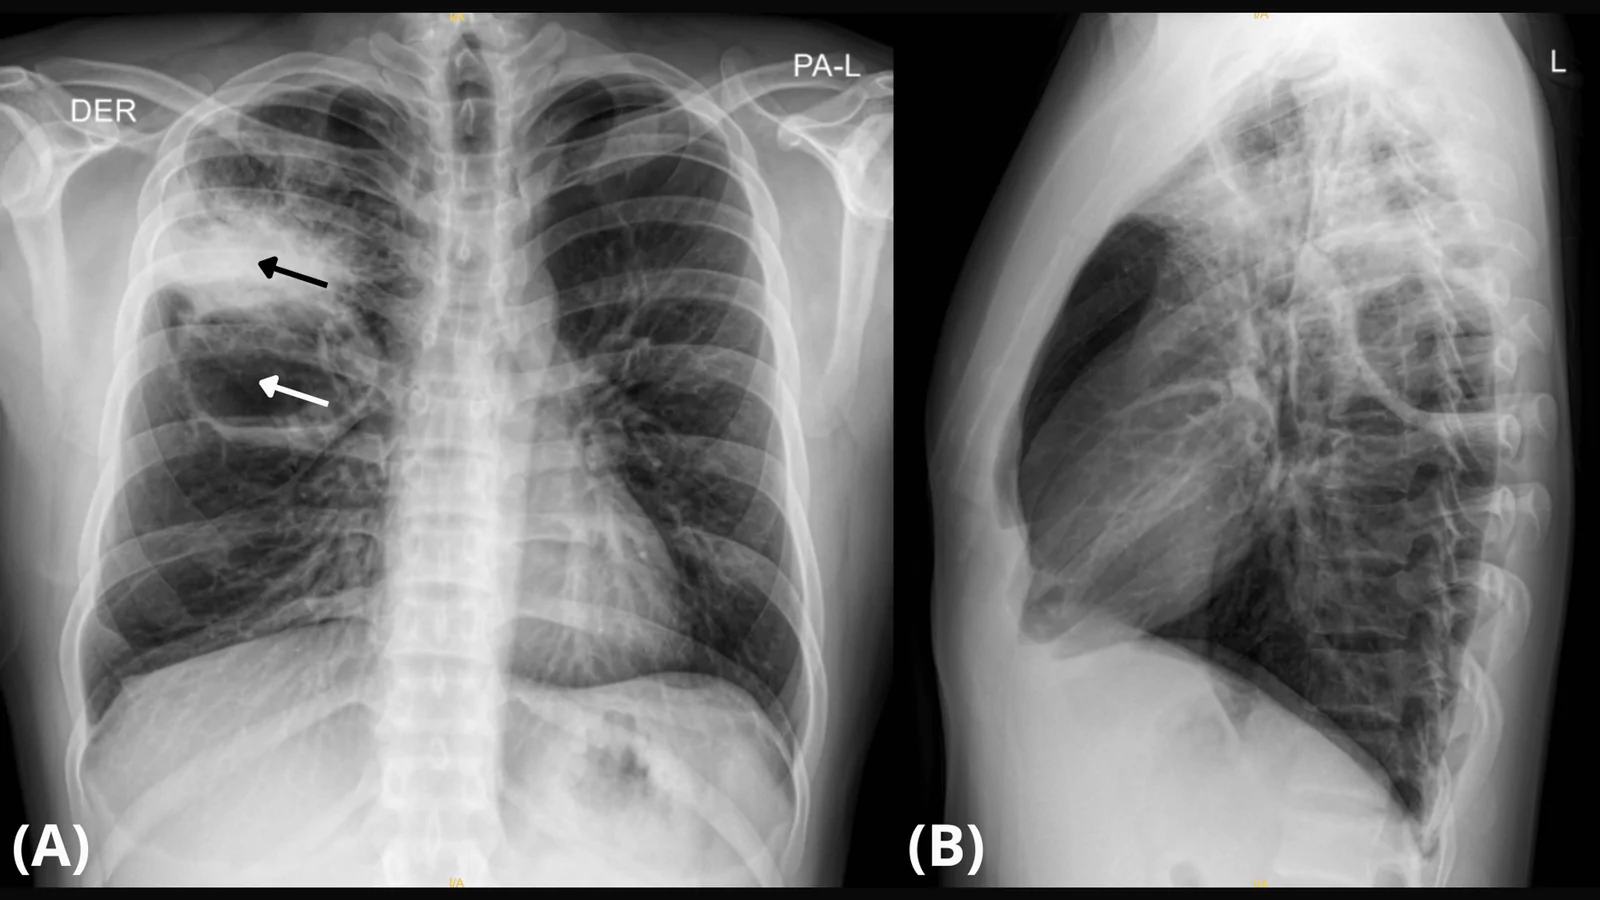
Posteroanterior and lateral chest radiographs on admission. (A) Posteroanterior chest radiograph showing right upper lobe consolidation (black arrow) overlying a radiolucent, cystic-appearing lesion (white arrow). (B) Corresponding lateral chest radiograph.
